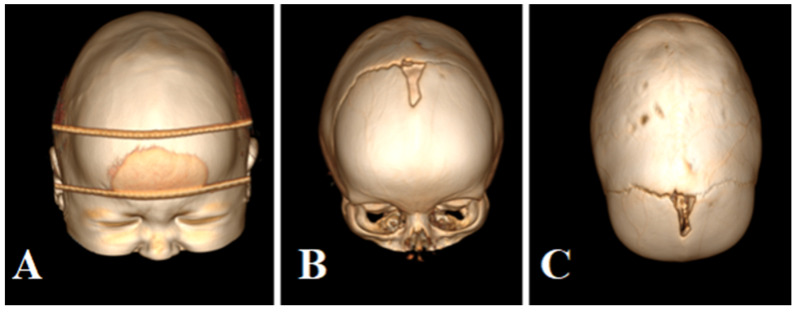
Patient’s CT scan before the surgery. (A) Frontal view with head tape fixation for CT examination, (B) frontal view with skull reconstruction, (C) top view with skull reconstruction.
